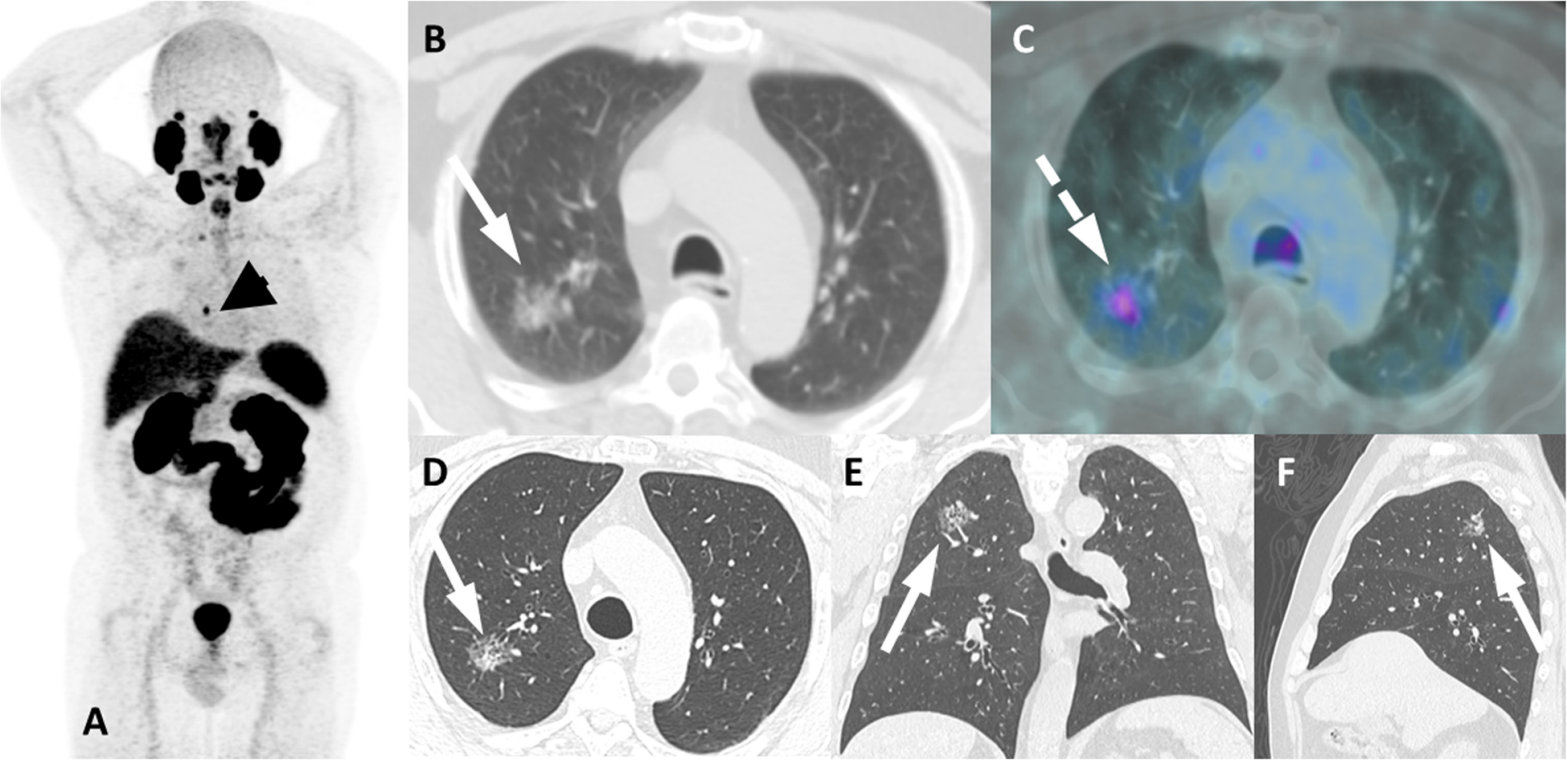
Lung adenocarcinoma with PSMA uptake. ^68^Ga-PSMA-PET MIP (a), axial CT (b) and axial PET/CT (c) and axial, coronal and sagittal dedicated lung CT (d, e and f) show a slight uptake (dashed arrow) in peribronchial subsolid pulmonary nodule in the posterior segment of the right upper lobe (arrows), which biopsy revealed primary lung adenocarcinoma. Note also a confirmed prostate cancer metastatic right pulmonary hilar lymph node with intense uptake (arrowhead in A)
